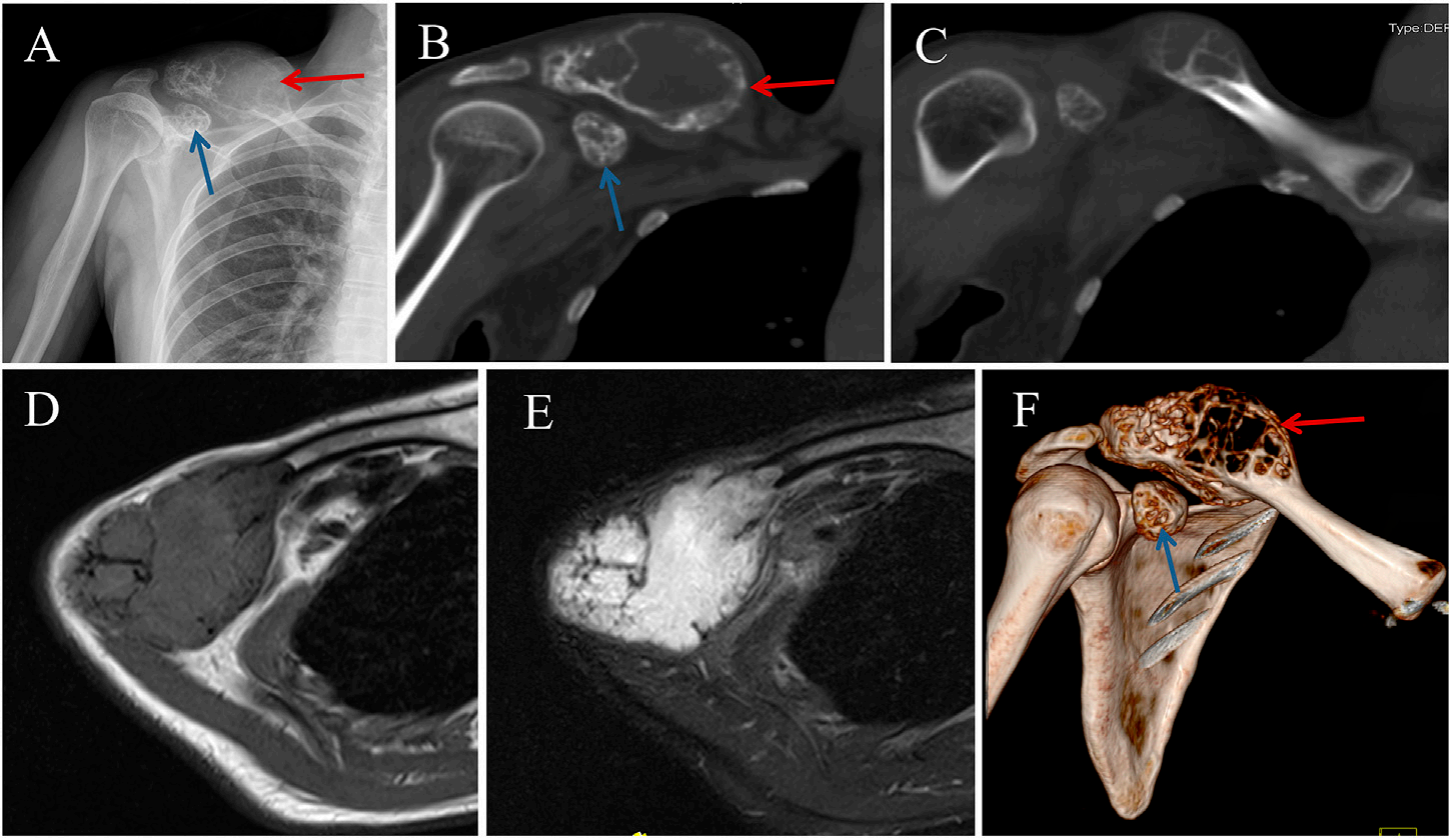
Preoperative image data of the patient. (A) Anteroposterior X-ray of the right clavicle showing an large and expansive lesion (Red Arrow), with another lesion involving coracoid process (Blue Arrow). (B, C) Computed tomography (CT) images showing osteolytic lesions with cortex-destructing. Magnetic resonance imaging showing the expansive lump with isointensity to muscle on a (D) T1-weighted image and homogeneous hyperintensity on a T2-weighted image (E). (F) 3D model of the shoulder reconstructed from CT scans.
